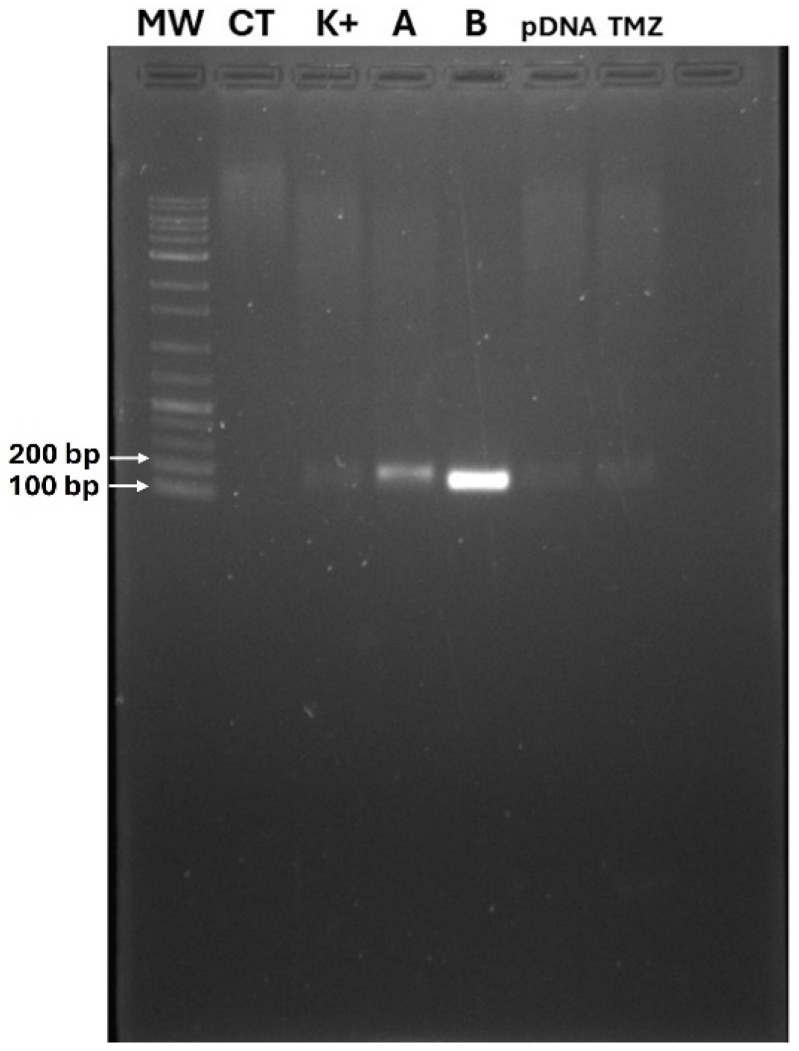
PCR analysis of p53 mRNA in U-87 cells after 24 h of transfection mediated by A—optimal BSA-free and B—BSA-coated TMZ-WRAP5/p53 complexes (1 µg of pDNA, N/P ratio = 1.03). MW—DNA ladder molecular weight marker; CT—control without cDNA sample; K+—untreated cells. Naked pDNA and TMZ alone were used as additional controls.
